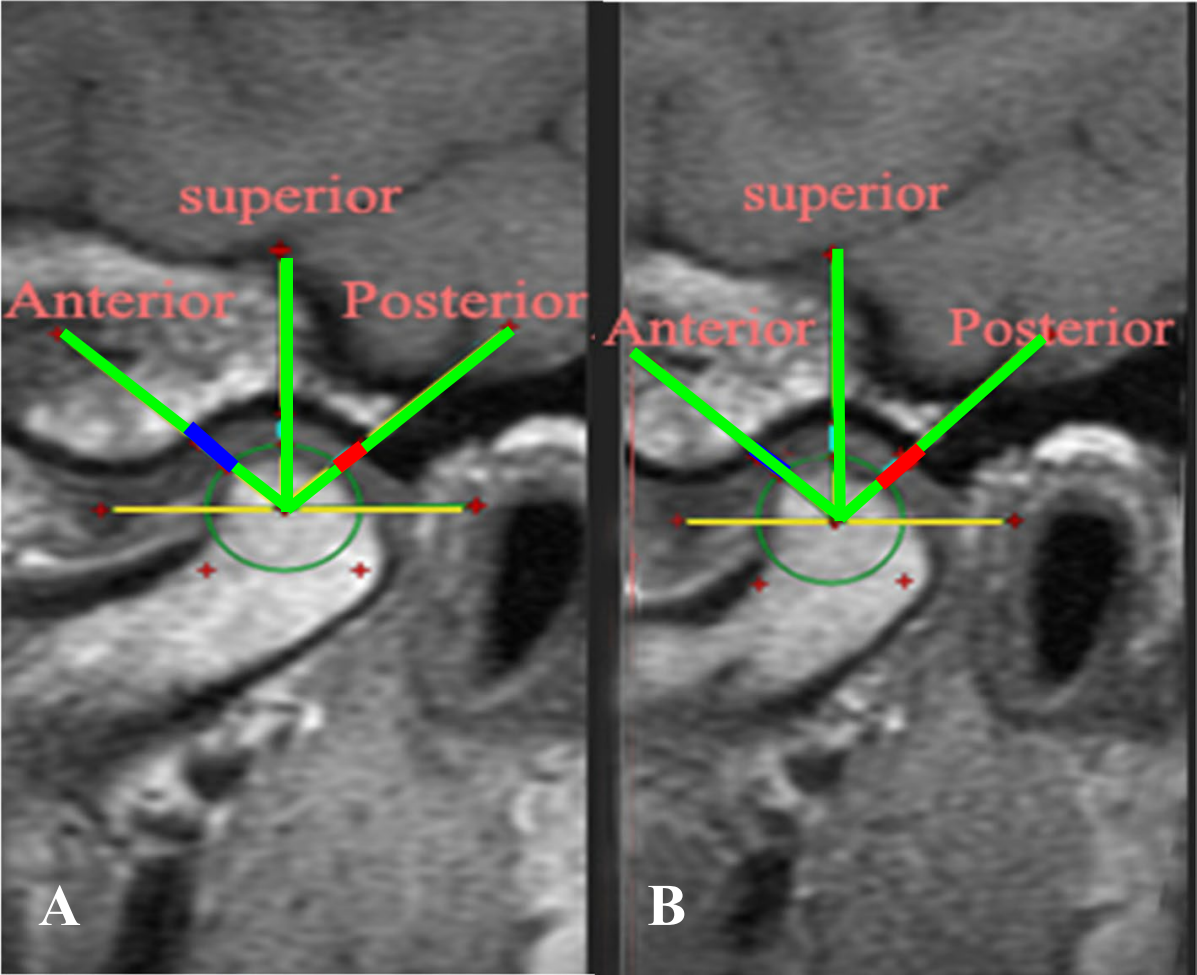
MRI showing the improvement in condylar position. A. Before BTX-A injection, B. After BTX-A injection
